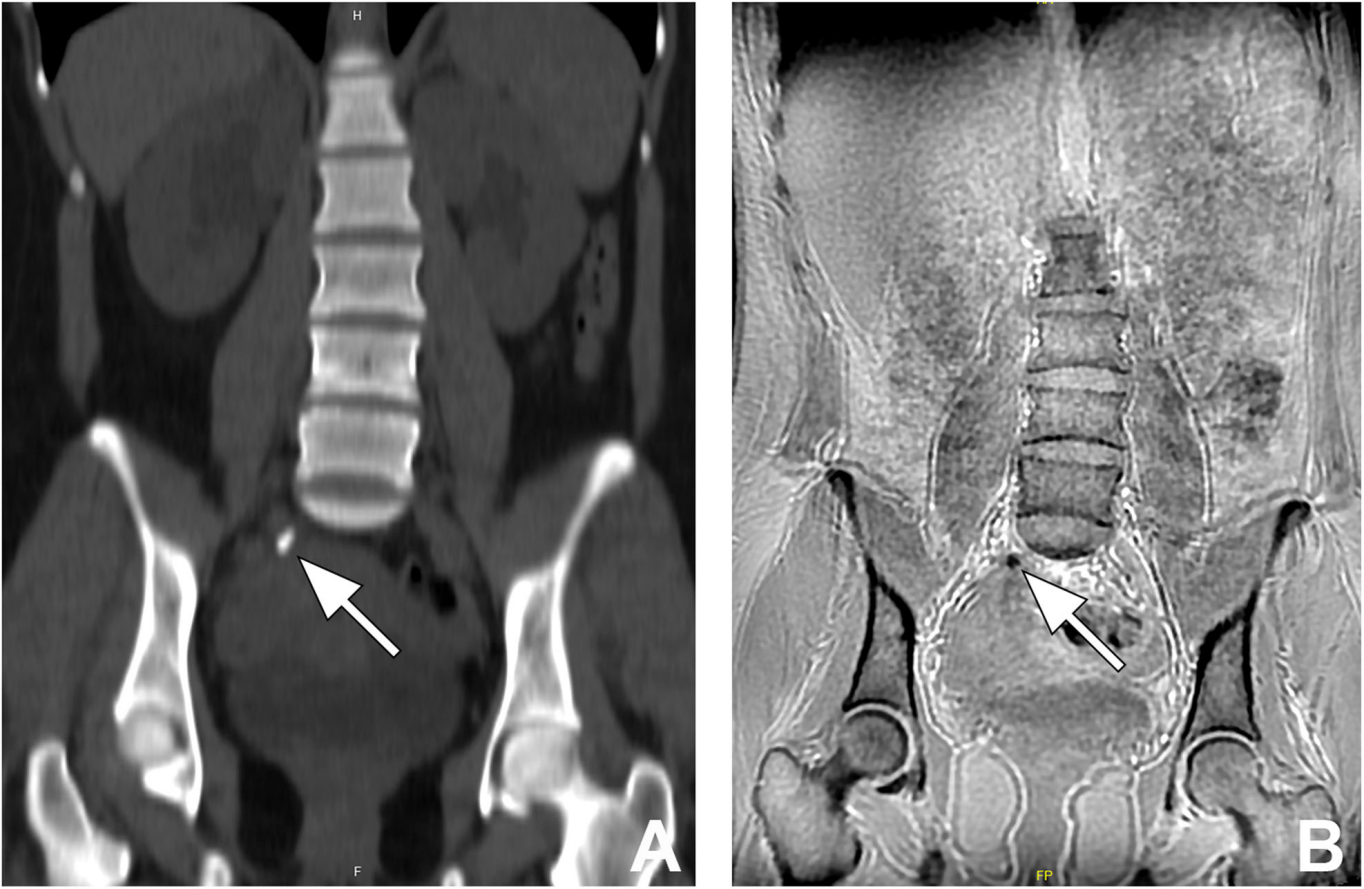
A 17-year-old girl with a right ureter stone. A Coronal non-enhanced CT image and (B) coronal ZTE-MR image at the same level show the stone (arrow) in the right ureter
